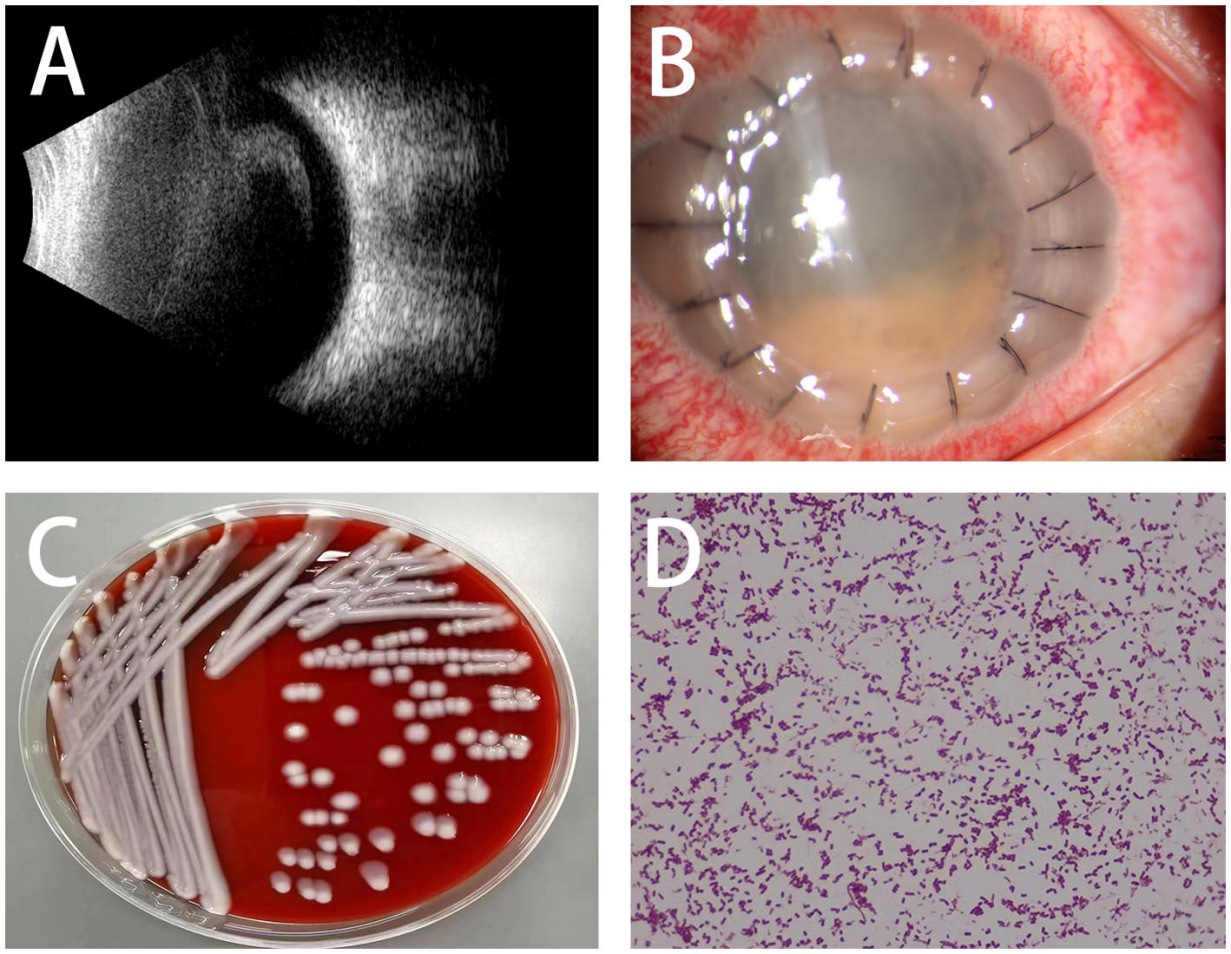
(A) Ultrasound shows an increase in echogenicity in the vitreous cavity. (B) Clinical picture shows whitish infiltrates in the center of the graft with a small hypopyon. (C,D) Bacterial cultures of corneal scrapings and the bacterial smear confirmed the carbapenem-resistant *Klebsiella Pneumoniae* infection.
